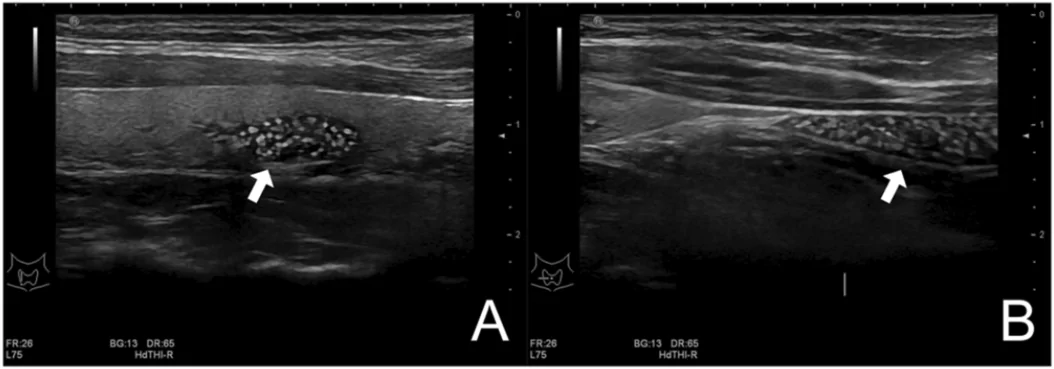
Ultrasound of the right thyroid lobe showing a 10 × 4 mm hypoechoic area with multiple fine hyperechoic foci (*starry‐sky* appearance), consistent with intrathyroidal thymic tissue; (A) comparative view of the thymus versus adjacent thyroid parenchyma; (B) longitudinal view of the intrathyroidal thymic tissue. This finding is relevant in the differential diagnosis, as intrathyroidal thymic tissue may mimic suspicious thyroid lesions on ultrasound in children.
